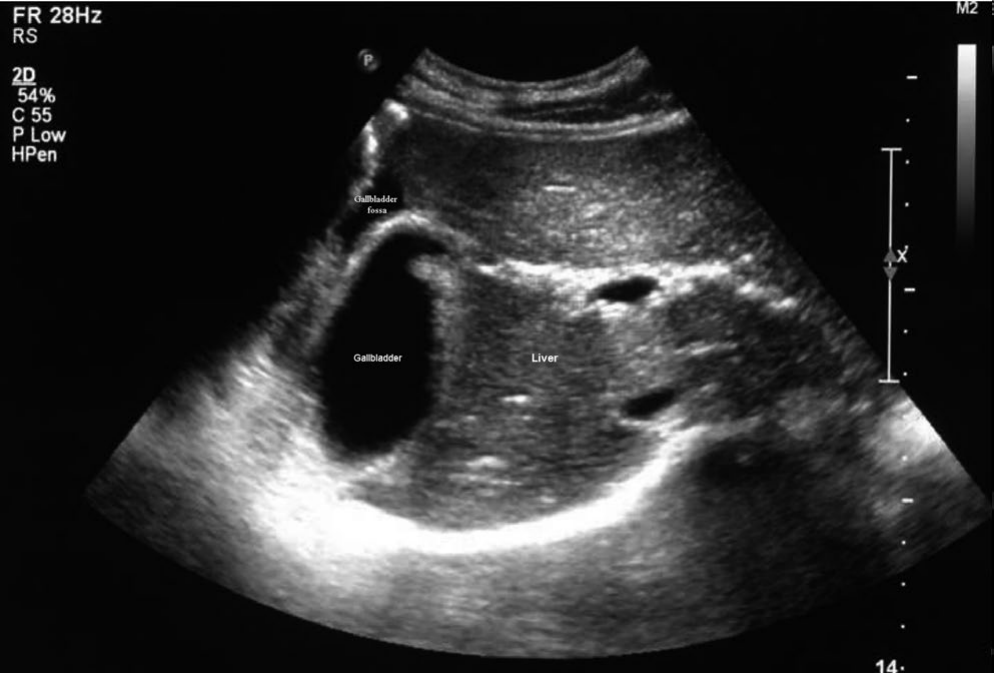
Ultrasound appearance of gallbladder volvulus
